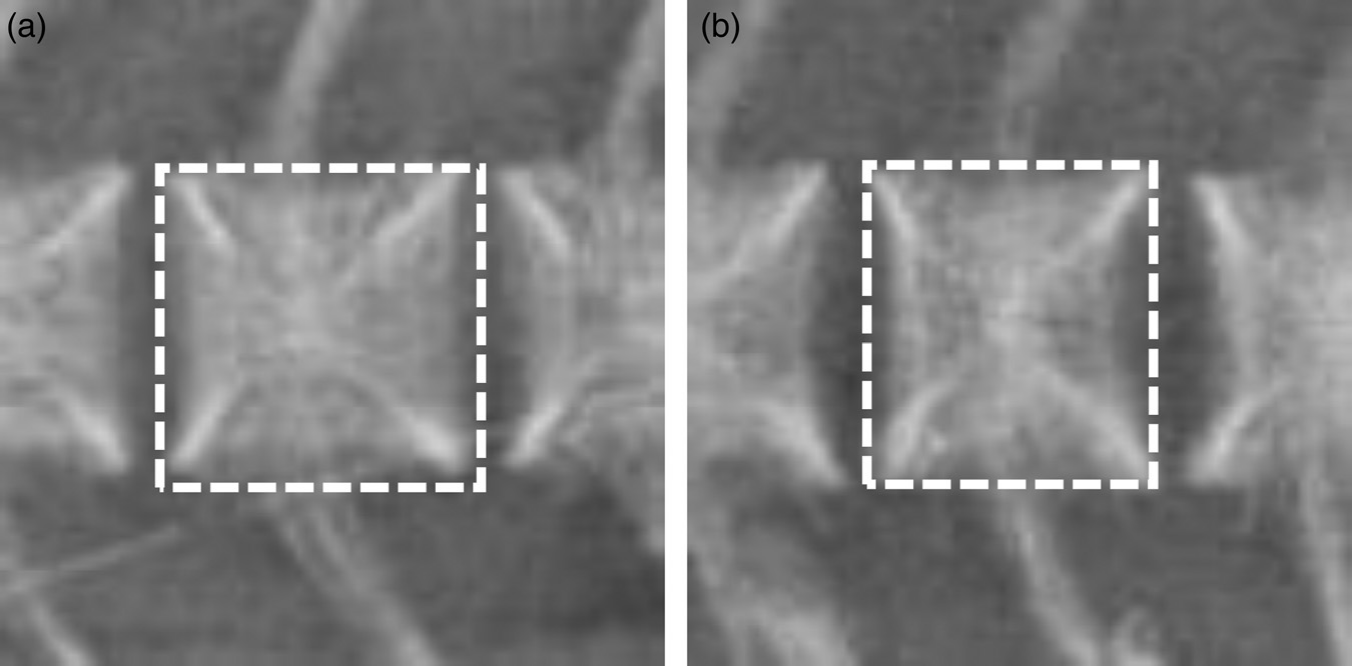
Radiography: detail of vertebrae with different length:height ratios. (a) Vertebra with normal proportions, length:height ratio about 1; (b) vertebra with reduced length, length:height ratio 0·92. Outlines of structures are indicated by dotted lines.
